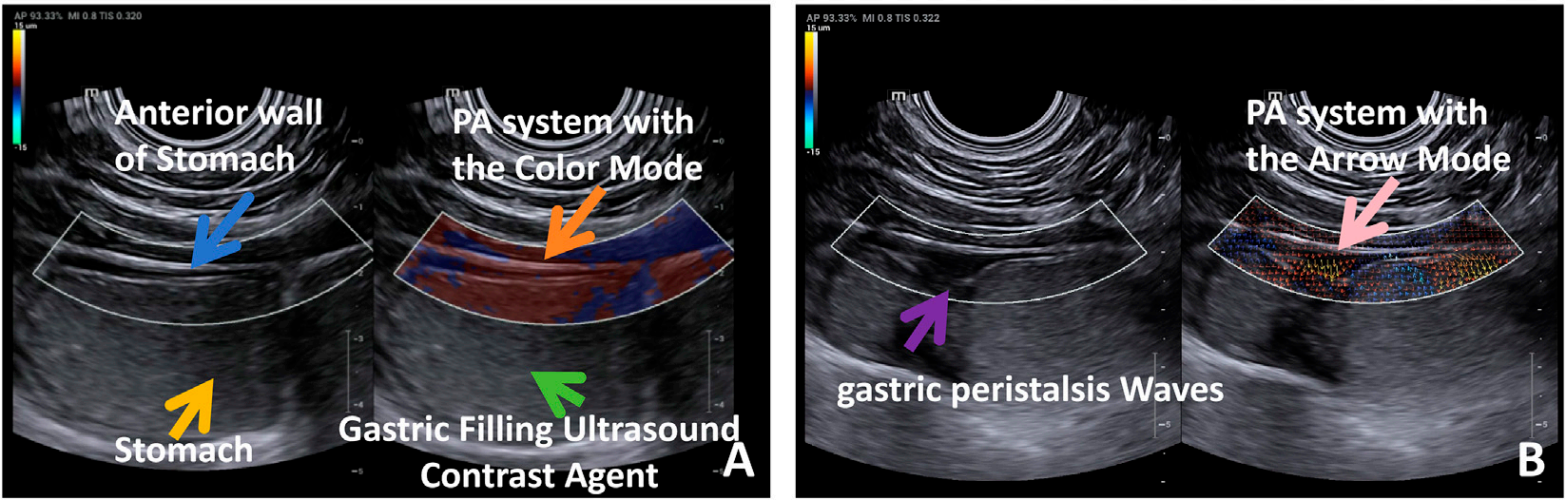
(A) Real-time PA imaging using Color Mode. The color-coded regions represent vertical displacement of gastric wall tissue. Red areas indicate upward motion (away from the probe), while blue areas indicate downward motion (toward the probe). The alternating red-blue waveforms reflect rhythmic peristaltic activity and propagation direction. Blue arrow: Anterior wall of Stomach; Orange arrow: PA system with the Color Mode; Yellow arrow: Stomach; Green arrow: Gastric Filling Ultrasound Contrast Agent. (B) Real-time PA imaging using Arrow Mode. The arrows represent tissue displacement vectors, where the direction of each arrow indicates the trajectory of motion and the length indicates displacement magnitude. Color and brightness encode vertical velocity, allowing visualization of dynamic propagation of peristaltic waves from proximal to distal stomach. Purple arrow: gastric peristalsis Waves; Pink arrow: PA system with the Arrow Mode.
